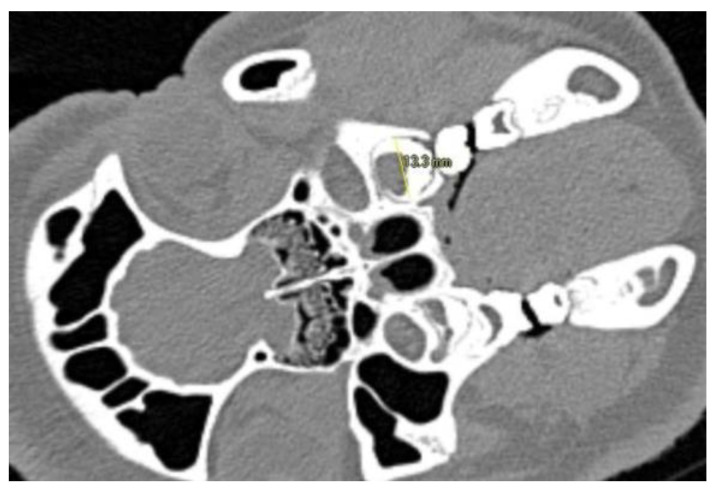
Visualization of the measuring procedure in CT-slices. Figure depicts measurement in buccal-oral axis. The outer contour of the tooth is determined as the interface between the hyperdense structure of the tooth and the hypodense structure of the desmodontal gap.
